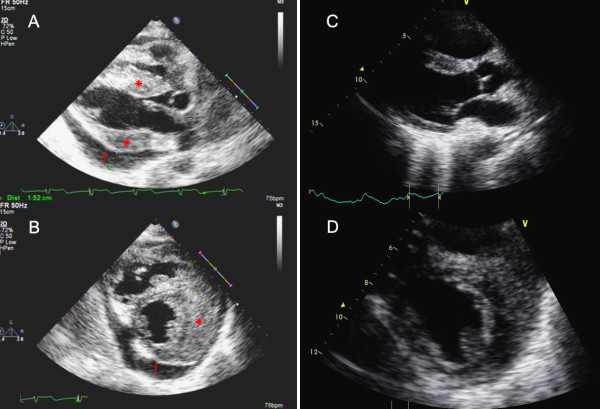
Echocardiography before treatment (left section, A: parasternal long axis and B:parasternal short axis) revealed heterogeneous infiltration involving the aortic root, and markedly increased left and right ventricular wall thickness (*). Moderate pericardial effusion (15.2 mm) was also noted (†). Six months after treatment (right section, C: parasternal long axis and D: parasternal short axis), reduced thickening of the myocardium and resolution of pericardial effusion were noted.
